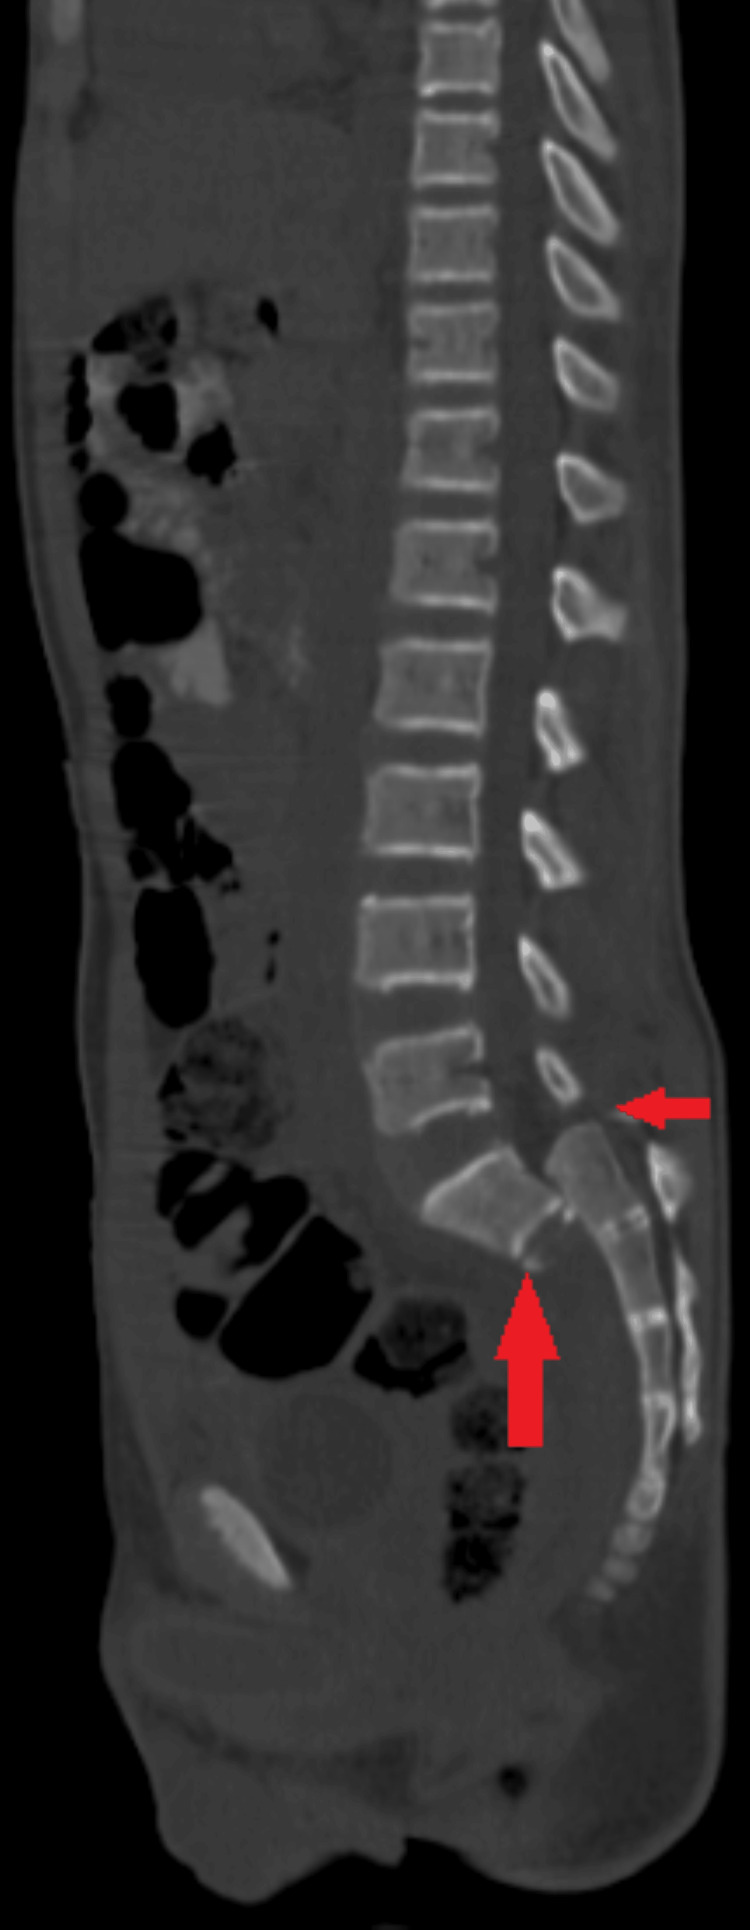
CT lumbar spine sagittal sequence demonstrating spondyloptosis at the L5-S1 level
